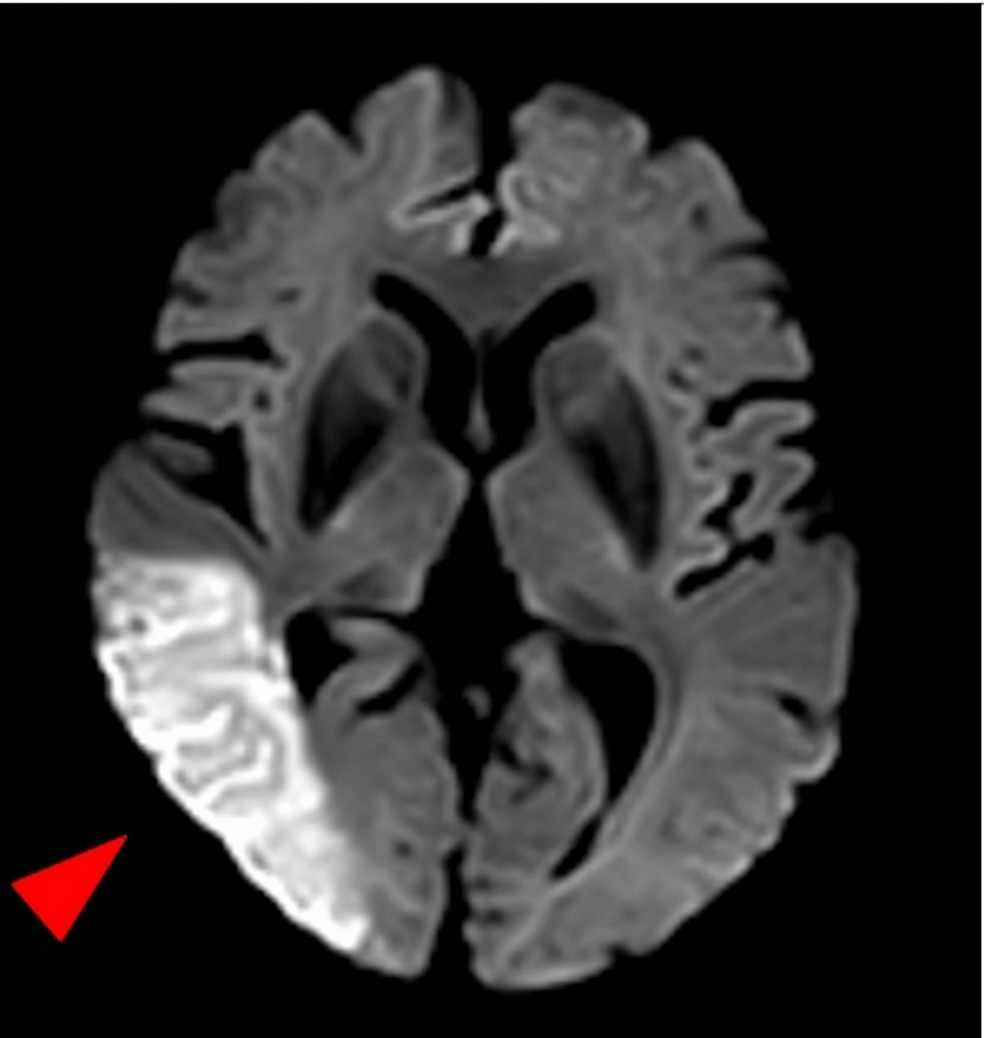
Head MRI showing high intensity in the right occipital lobe on diffusion-weighted imaging (arrow)
